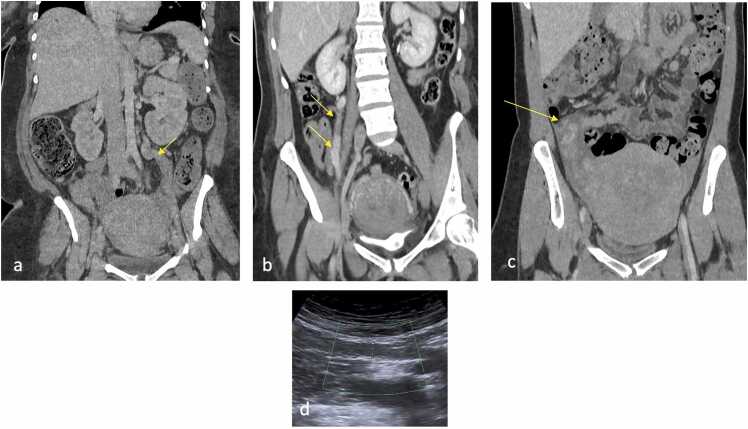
a, b): Coronal CECT demonstrating normal prominent ovarian veins. Note the smooth contours and no surrounding inflammatory stranding (arrows). DIAGNOSIS: Normal c) Coronal CECT demonstrated ovarian vein thrombosis. The right ovarian vein is engorged and inflamed with surrounding free fluid (arrow). d) Doppler US demonstrating absence of flow in the right ovarian vein consistent with thrombosis. DIAGNOSIS: Ovarian vein thrombosis.
